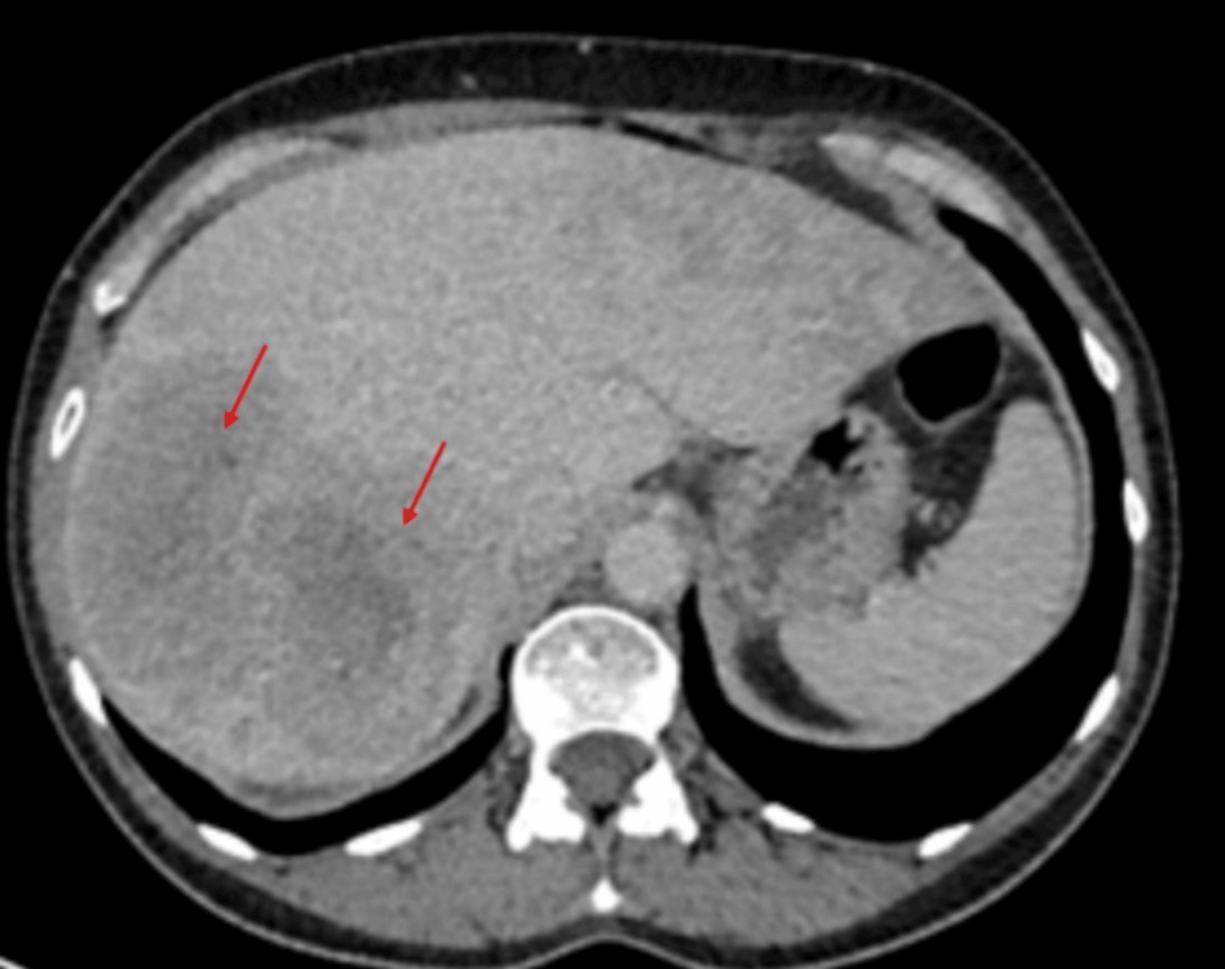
Initial CT of the thorax/abdomen/pelvis on 28 August 2024 showing multiple liver lesions (red arrows).
